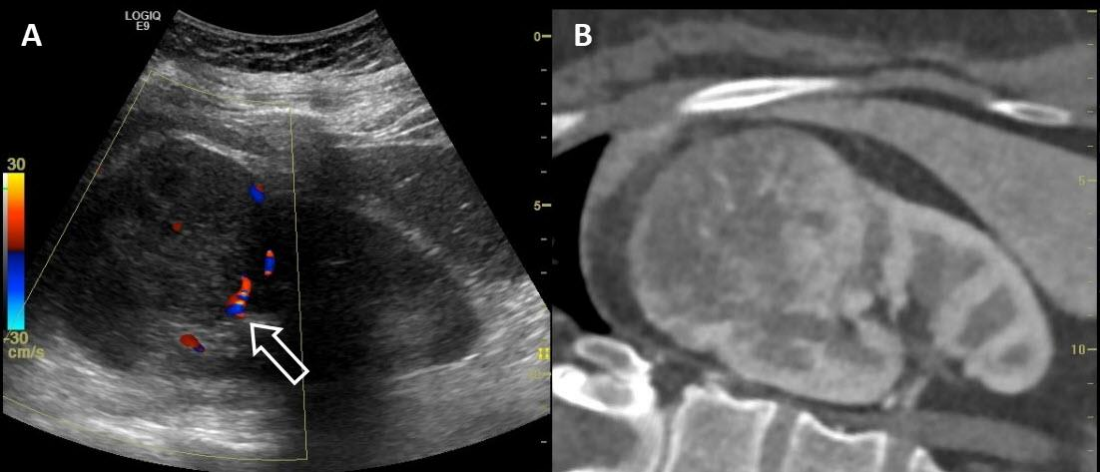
Ultrasound Computed Tomography (US-CT) Fusion US-CT fusion side by side: US color doppler (A) and CT images (B) of a US-CT fusion study. Longitudinal view of the kidney showing a space-occupying lesion in the upper pole of the kidney. Fusion imaging allows real-time assessment of tumor blood flow (arrow).
